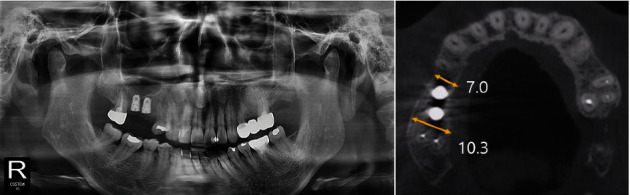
Post-implantation radiograph views (Case 3). The width of the alveolar bone was well-maintained, and the implant was placed.
